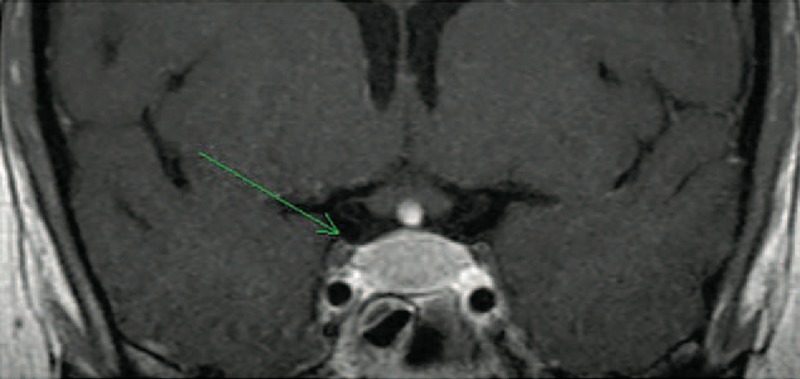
Patient 3 in 2007: coronal brain T1-weighted MR image after intravenous administration of gadolinium showing an enlargement and intense enhancement of pituitary.
